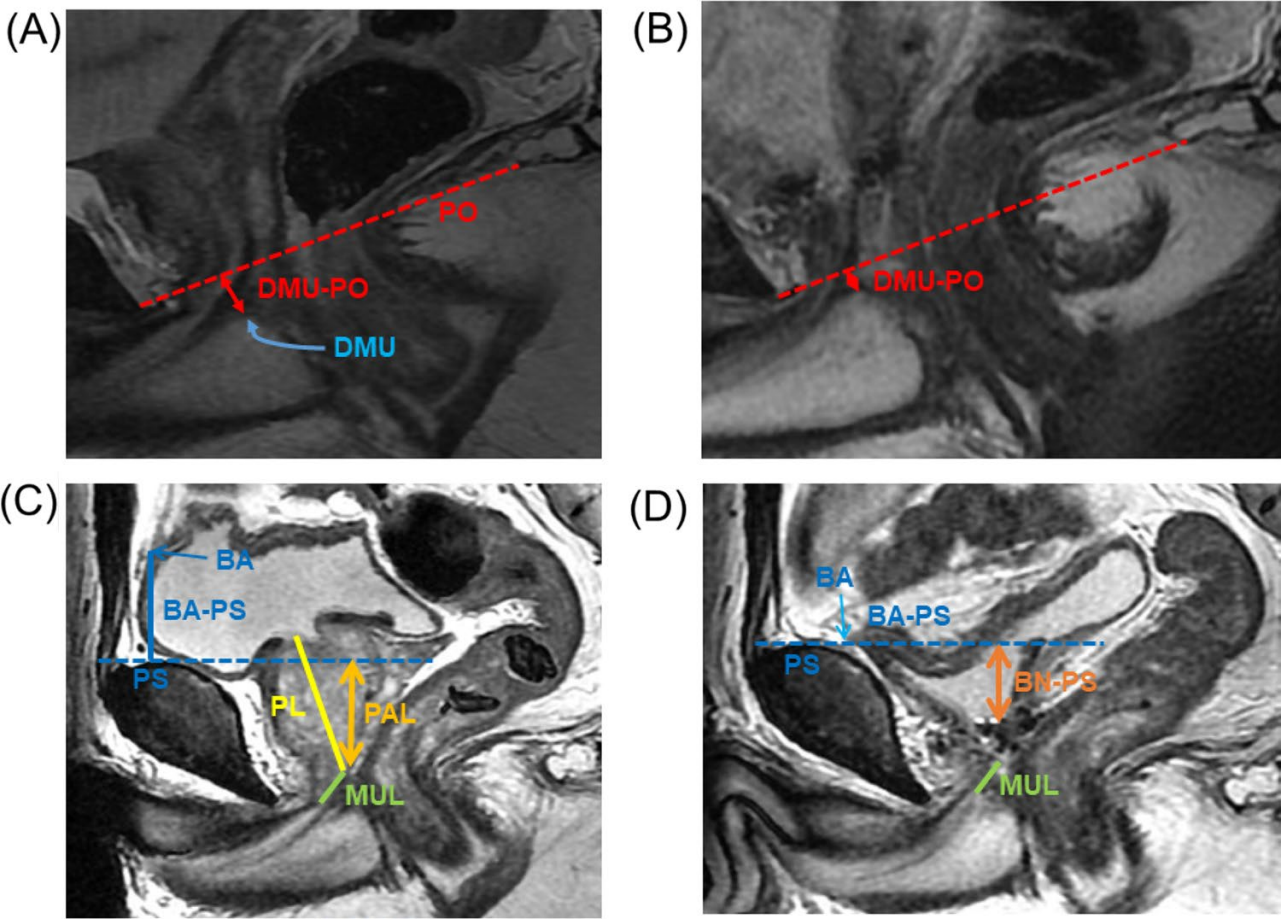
Parameters on mid-sagittal magnetic resonance imaging before (A, C) and 10 days after (B, D) robot-assisted radical prostatectomy. (A, B) The pelvic outlet (PO) (dashed red line) is defined as the line between the lowest end of the pubic bone and the tip of the coccyx. DMU-PO (red bidirectional arrowheads) is the distance from the distal end of the membranous urethra (DMU) to the midline of the PO. (C, D) Bladder attachment (BA) is defined as the upper edge of the attachment between the anterior bladder wall and abdominal wall. Pubic symphysis (PS) is defined as the upper edge of the PS. BA-PS (blue line) is the distance from the BA to the PS. Prostate length (PL) (yellow line). PS-to-prostate apex length (PAL) (orange bidirectional arrowheads) is defined as the distance between the extension lines of the suprapubic ridge line (dashed blue line) and the prostate apically. Membranous urethral length (MUL) (green line). Bladder neck to PS (BN-PS) (brown bidirectional arrowheads) is defined as the distance between the extension lines of the suprapubic ridge line and the lowest end of the bladder neck.
